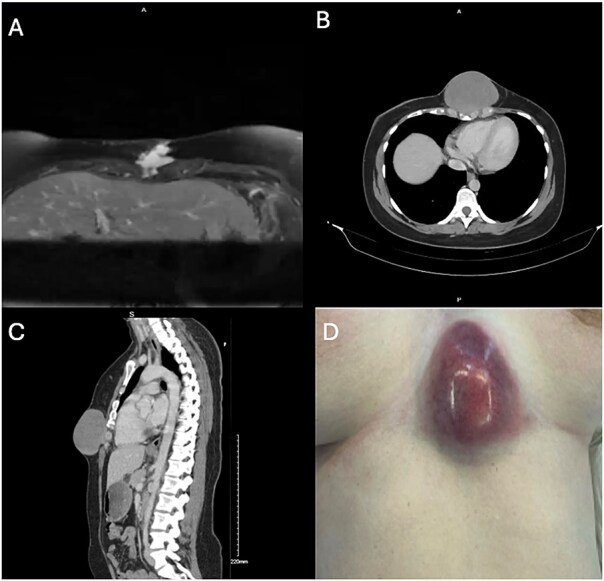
A: MRI axial plane. T2 weighted image. B, C: Non-contrast CT, axial and sagittal planes. D: Photograph of tumor at time of incisional biopsy.
